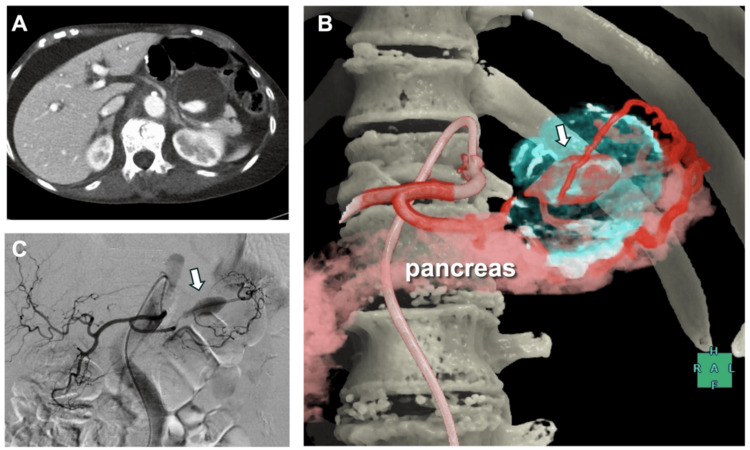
Imaging findings of the pancreatic tail pseudoaneurysm (A) Contrast-enhanced computed tomography (CT) showing a mass in the tail of the pancreas, consistent with a hematoma, with evidence of active contrast extravasation within the lesion. (B, C) CT-angiography (CTA) and digital subtraction angiography (DSA) revealing a pseudoaneurysm arising from the main trunk of the splenic artery (white arrow). Note the development of collateral circulation from the dorsal pancreatic artery to the splenic hilum through the transverse pancreatic artery.
